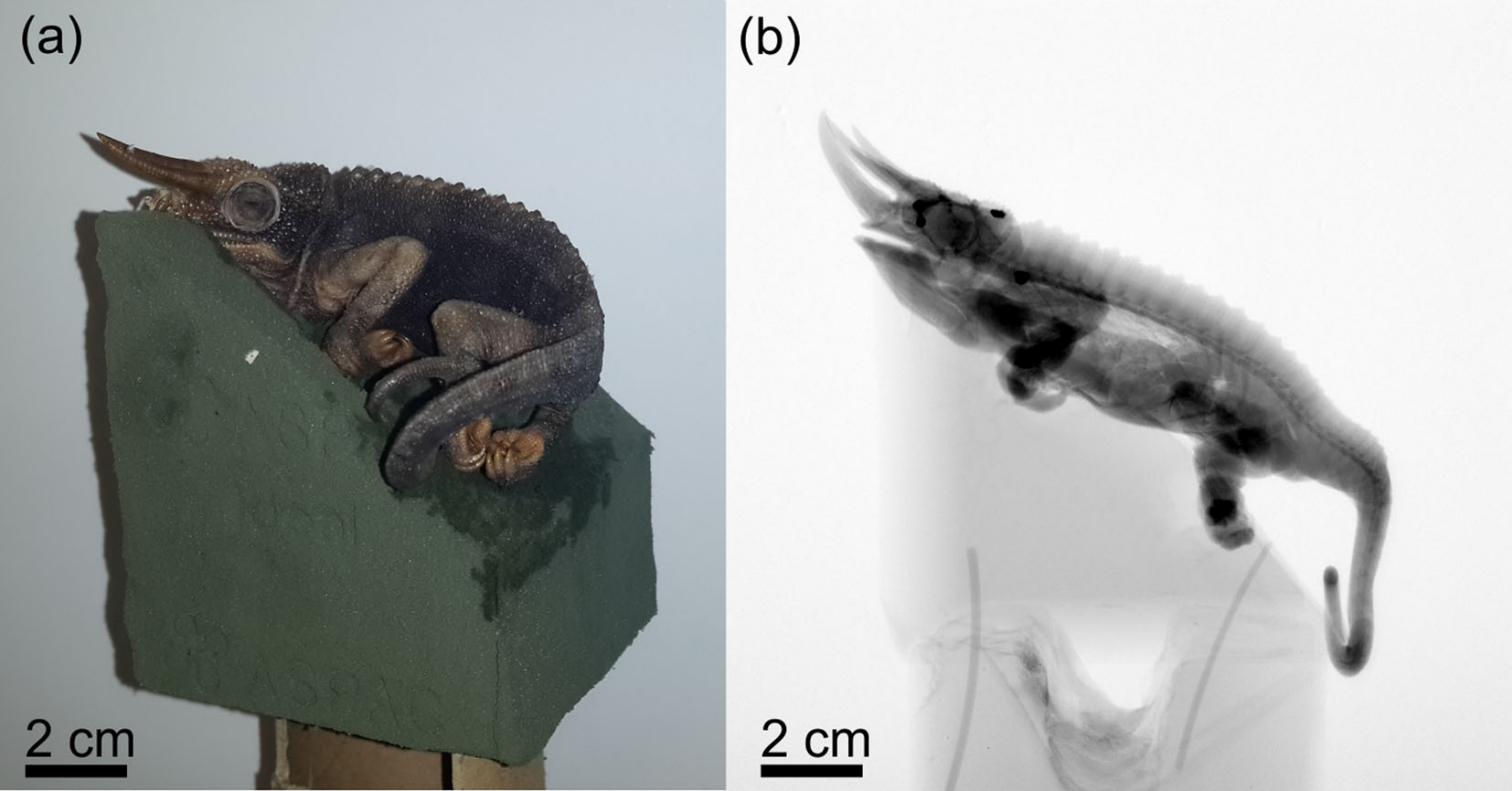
Mounting of a Jackson's chameleon. Florist foam mounting material forms the basis onto which the sample is placed (A). A 2D x-ray projection image shows the very low density of the mounting material (B).
